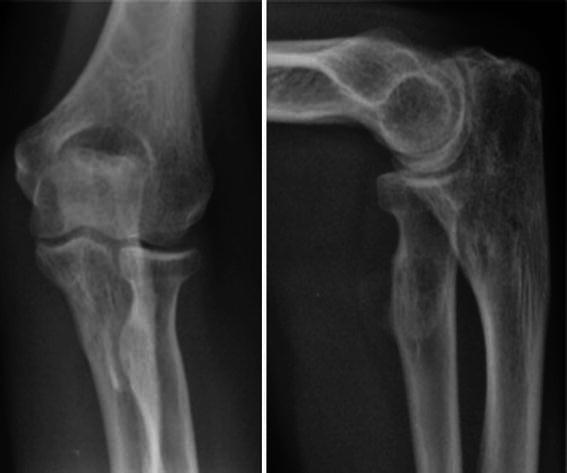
Radiographs at final follow-up showed good fracture healing and regression of heterotopic ossification
